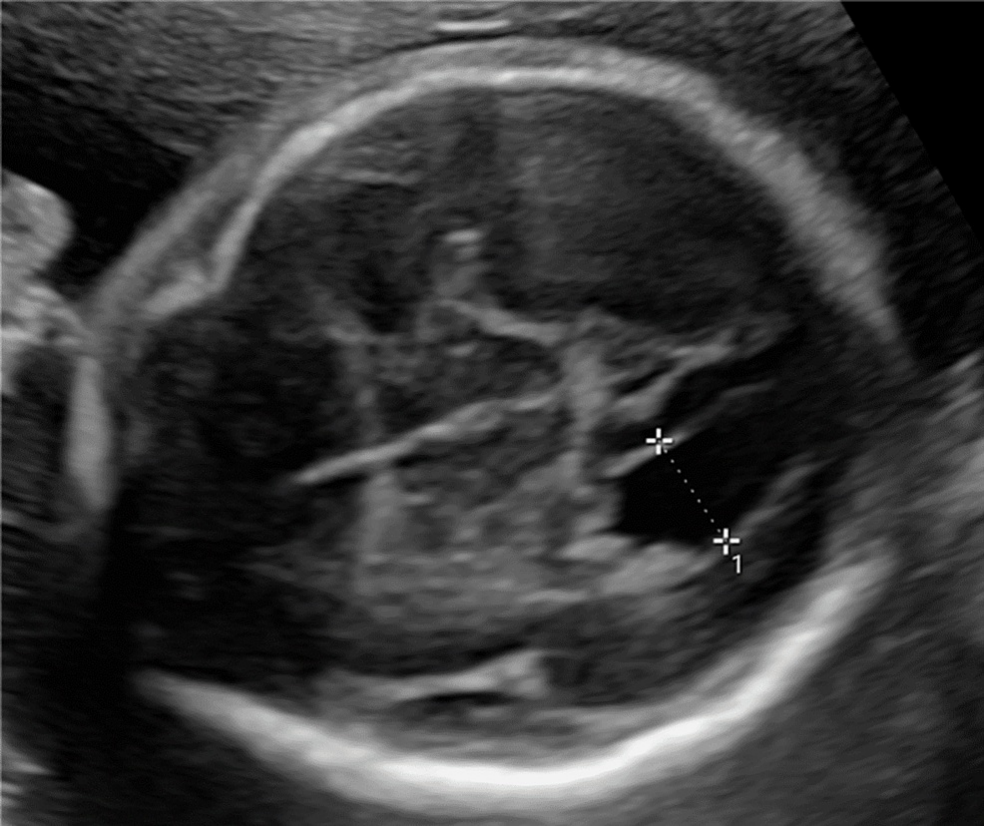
The 2D sonographic image of the fetal head (horizontal section) Mild enlarged atrial width (caliper, 11mm) is shown
